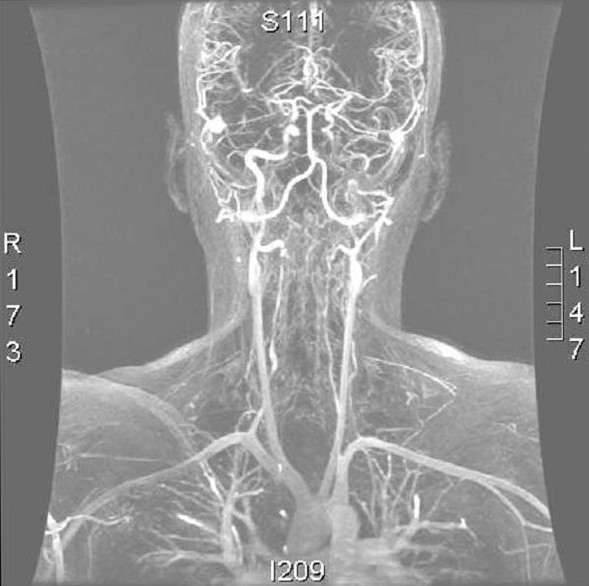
Angio-MRI (coronal section) of the supra-aortic vessels after injection of 20 cc of gadolinium in a woman aged 24 with EDS type IV, revealing a dissecting haematoma of the left internal carotid (black arrow), a bilateral dissection of the vertebral arteries in their V1 and V2 segments (white arrows) and a dissection of the middle and distal third of the right subclavian artery (head of arrow).
